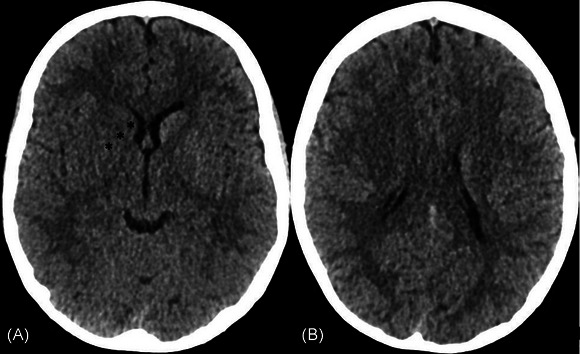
Axial noncontrast CT images at the level of the insula (A) and centrum semiovale (B) in a 12‐year‐old with right MCA M1 segment occlusion before undergoing mechanical thrombectomy. Established infarct is seen in the right caudate head, internal capsule, and lentiform nucleus (black asterisks). Baseline ASPECTS was 7. Pediatric mRS was 3 at 3 months and 2 at 9 months. ASPECTS indicates Alberta Stroke Program Early Computed Tomography Score; CT, computed tomography; MCA, middle cerebral artery; and mRS, modified Rankin scale.
